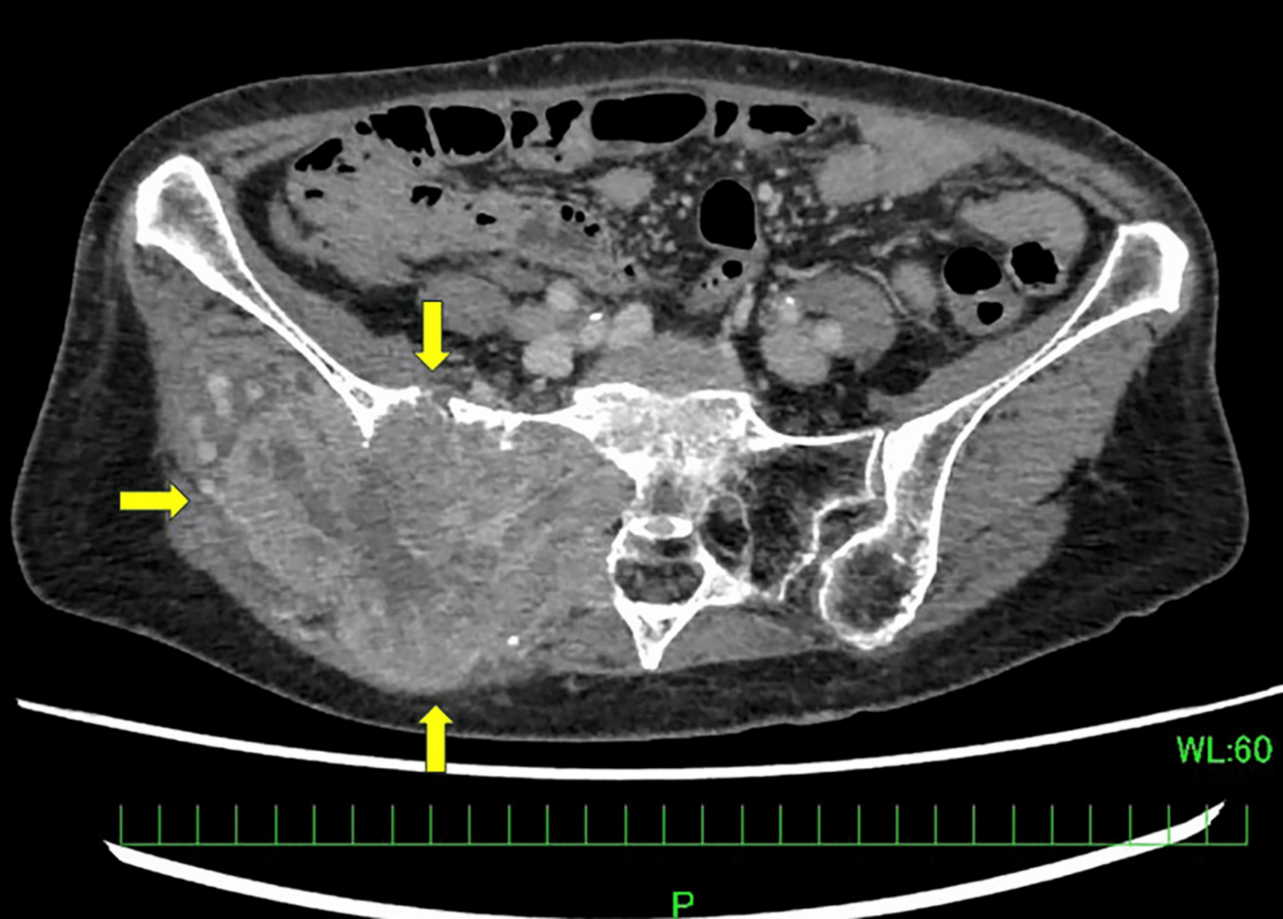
Contrast-enhanced computed tomography (CT) showing a right pelvic bone metastasis (arrows).
